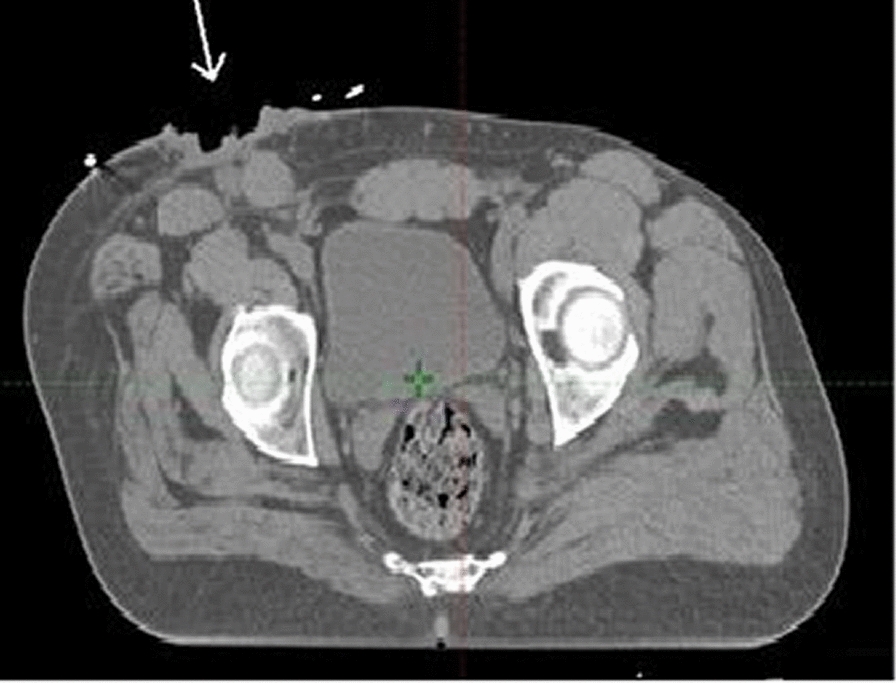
A pelvic cross-sectional computed tomography scan image showing an ulcerated right inguinal nodal mass lesions of about 83 mm × 40 mm (white arrow) infiltrating the anterior muscle complex with other multiple enlarged ipsilateral inguinal lymph nodes
